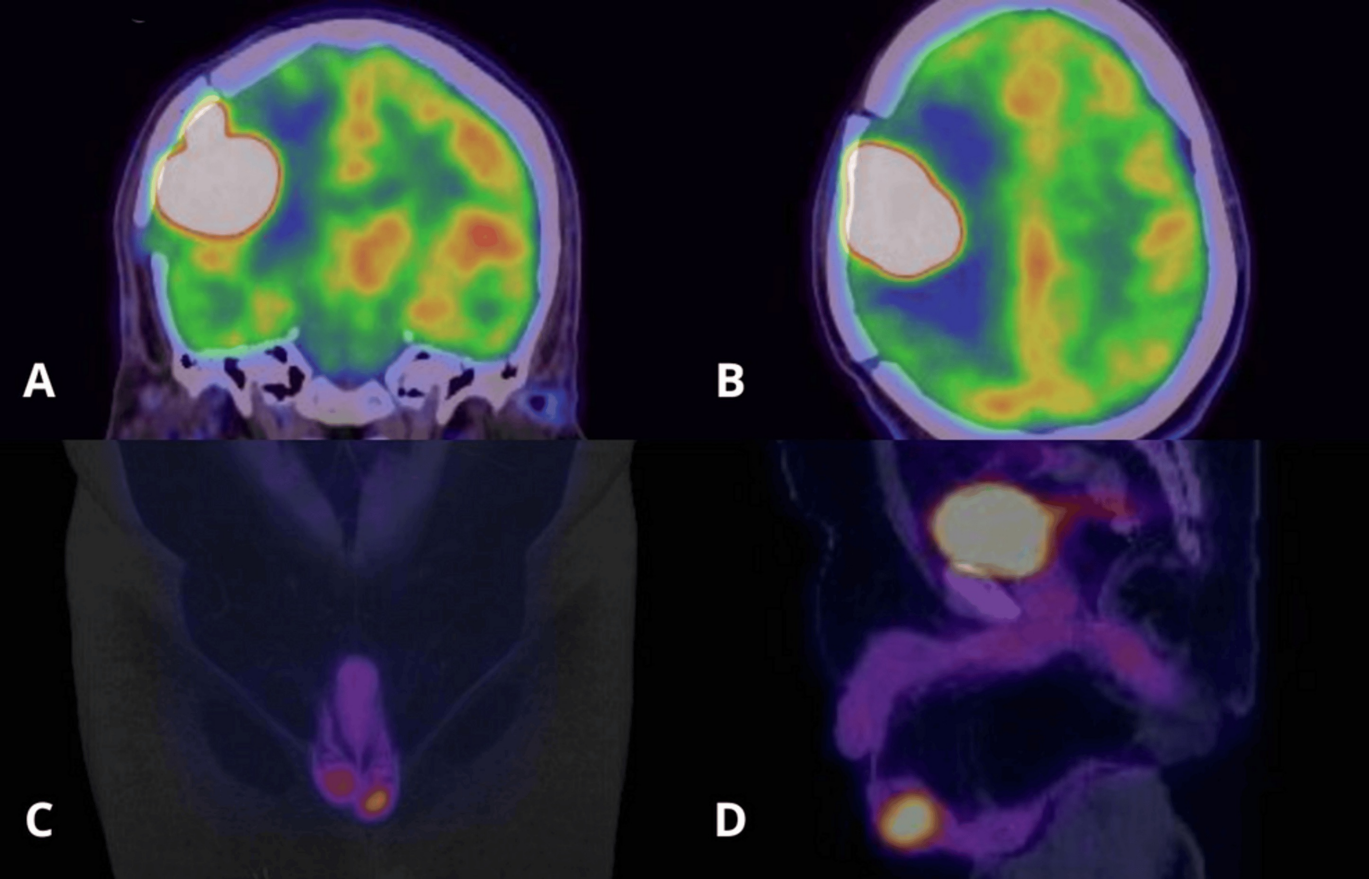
PET/CT A: coronal brain PET/CT post-craniotomy showing a hypermetabolic lesion located in the right frontoparietal region; B: axial brain PET/CT post-craniotomy showing a hypermetabolic lesion located in the right frontoparietal region; C: coronal abdominopelvic PET/CT showing hypermetabolic lesions located in the right and left testes; D: sagittal abdominopelvic PET/CT showing hypermetabolic lesions located in the right and left testes and the prostate.
